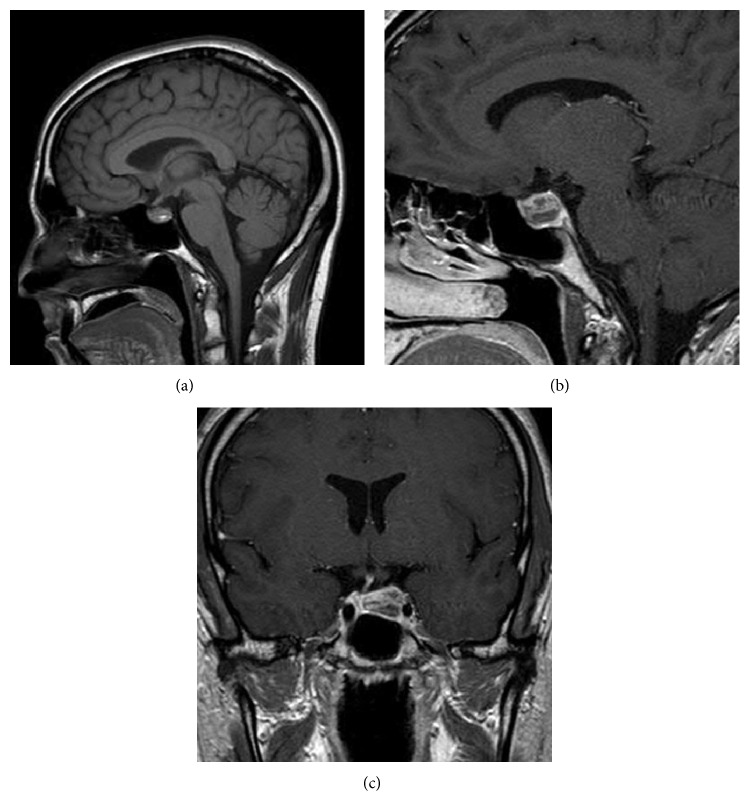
One week after the MRI in Figure 1, MRI revealed a shrinking sellar lesion on (a) sagittal precontrast T1-weighted, (b) sagittal postcontrast T1-weighted, and (c) coronal T1-weighted images. The enhancement and heterogeneity of the pituitary mass suggest hemorrhage. The optic chiasm is now visible in the coronal T1-weighted image. The pituitary stalk can also be seen deviated to the right.
